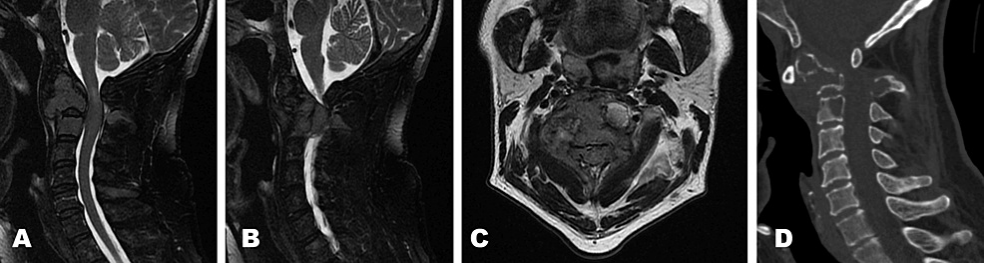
Sagittal (A, B) and axial (C) T2 sequence MRI shows vertebral bodies with heterogeneous signal and uptake throughout the entire spine secondary to multiple myeloma, with greater involvement at levels C1, C2, and C3. In this last segment, the infiltrative tissue involves adjacent paravertebral tissues and extends into the spinal canal, causing a narrow canal and compressing the adjacent spinal cord (C), which shows an increased signal, findings consistent with compression myelopathy. There is involvement of foramina and nerve roots bilaterally at levels C1-C2 and C2-C3. It presents as an osteodestructive lesion in the CT scan (D).
